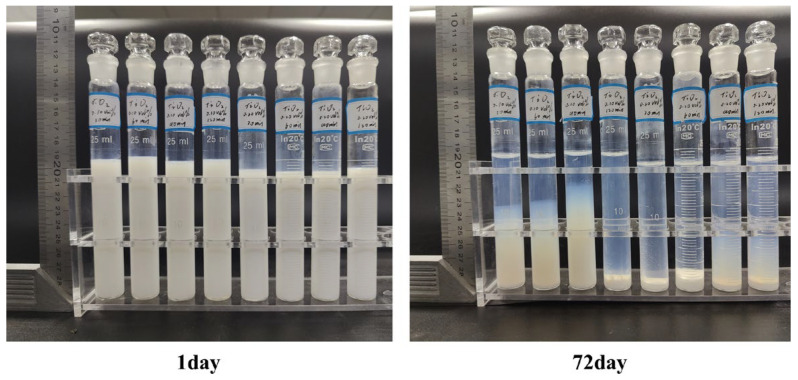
Sedimentation coefficient observation method for TiO_2_ nanofluids under identical surfactant conditions (0.15 wt% CMC) at different concentrations (0.10 vol% and 0.20 vol%) and varying ultrasonication durations (30–120 min).
